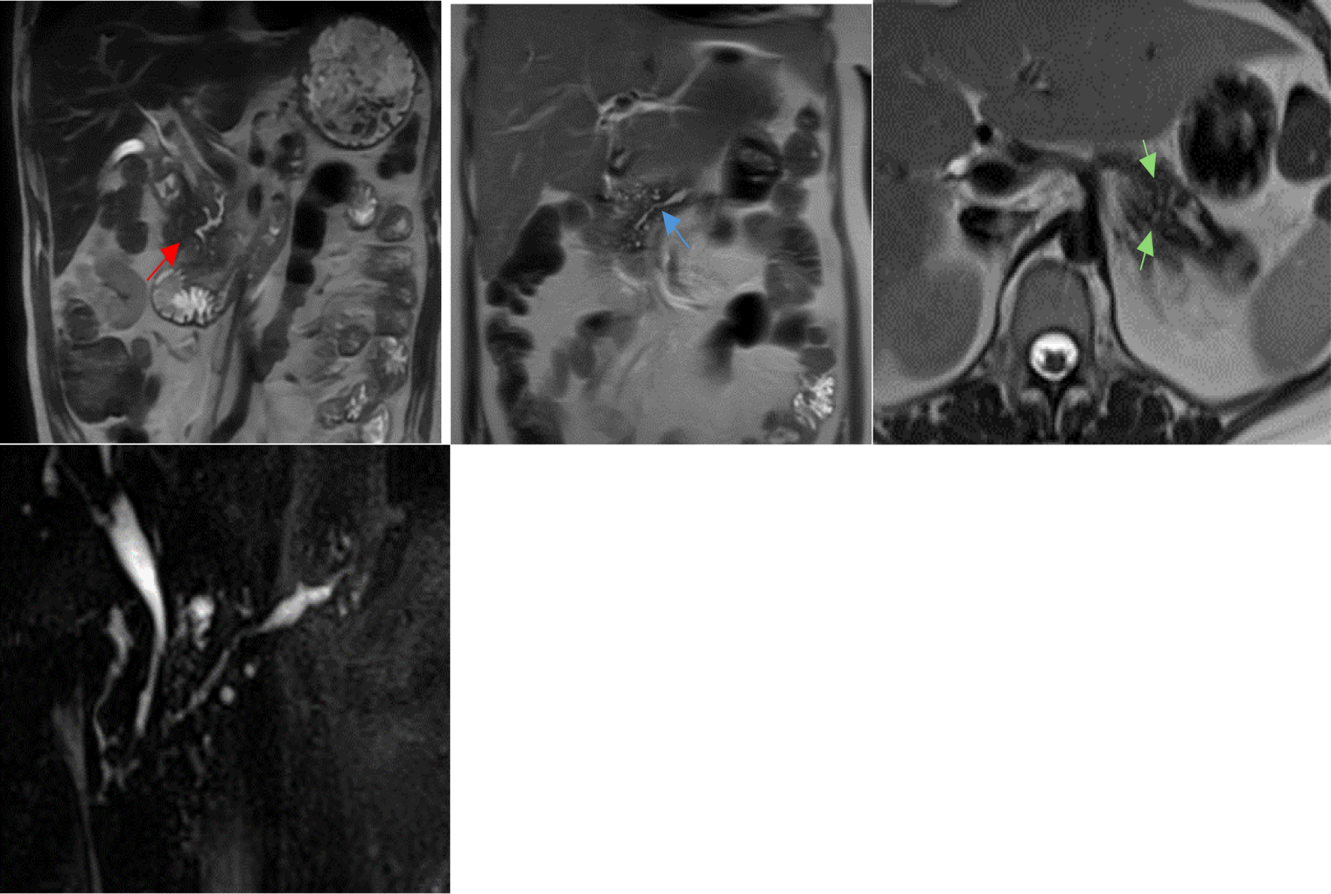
MRI (coronal T2 and axial) and MRCP from two patients with crhonic pancreatitis, showing T2 hypointense pancreatic signal (red arrow), tortuosity of the main pancreatic duct (blue arrow), and numerous prominent side branches (green arrows)
